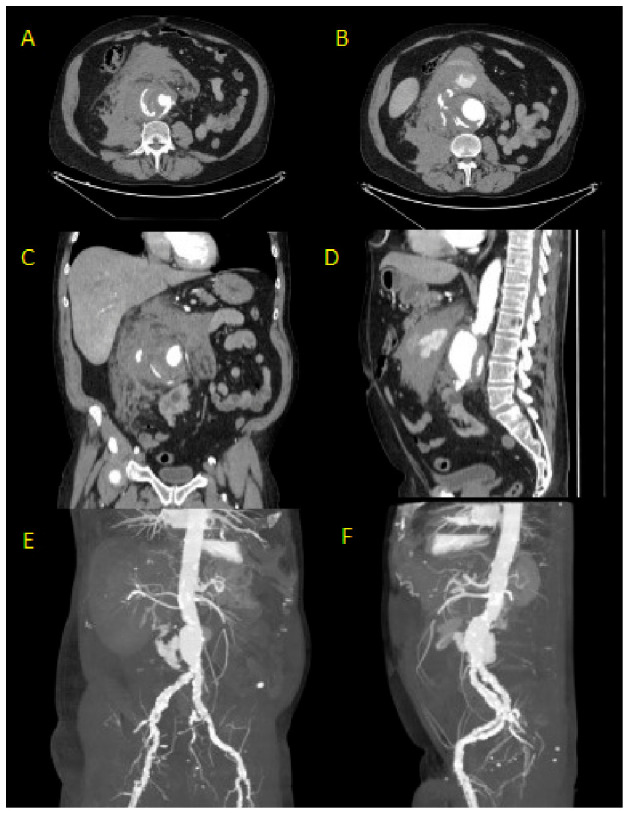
CT angiography ((A,B): axial at different level; (C): coronal; (D): sagittal and (E,F): vessel-window, VR image, in different position in rotation) showing a large dissected infrarenal abdominal aortic aneurysm with extensive mural thrombus and focal contrast extravasation. These features reflect advanced wall fragility and are highly suggestive of impending or active rupture, highlighting the role of CTA in emergency assessment and management.
